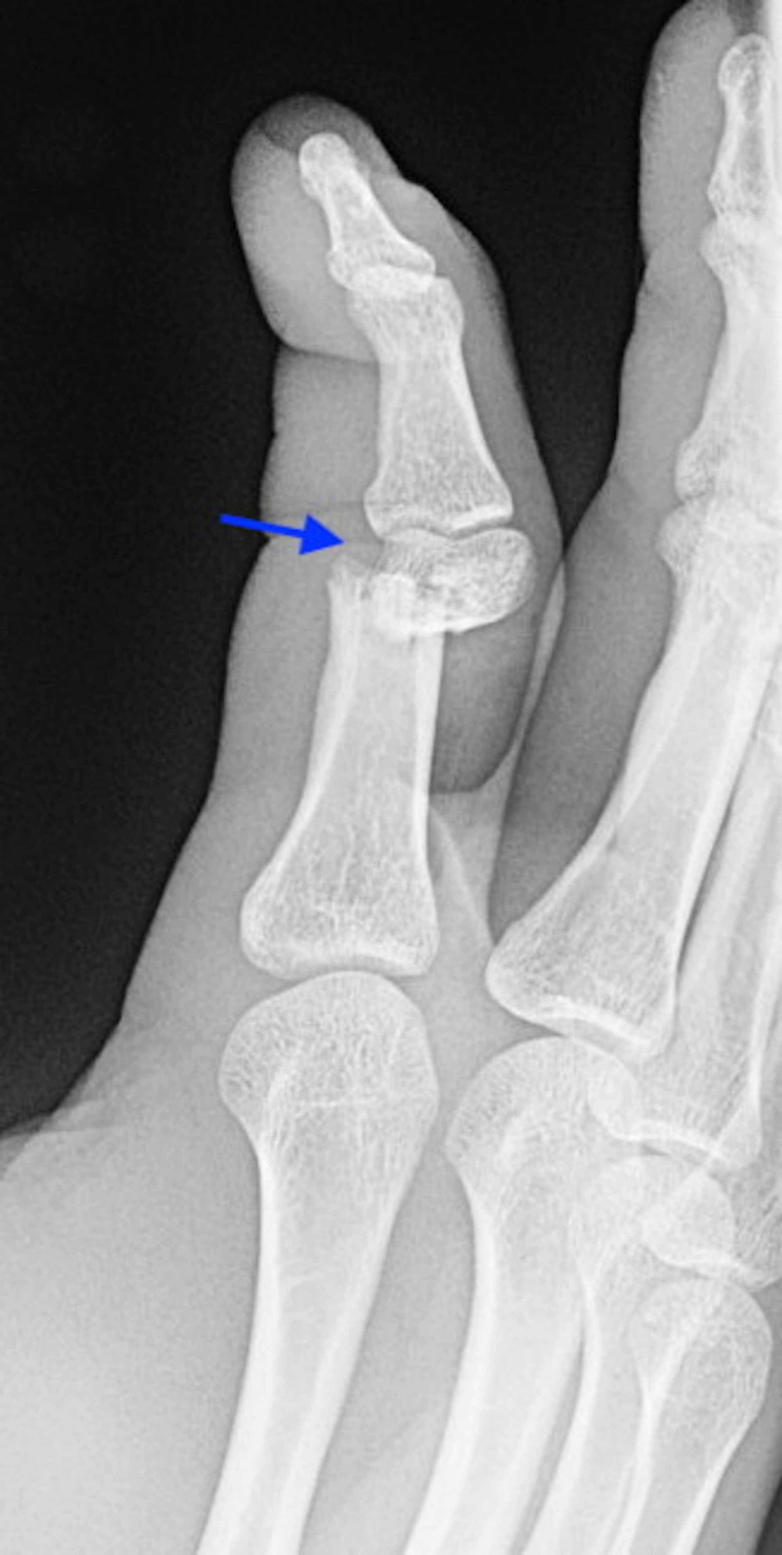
Oblique plain radiograph of the right index finger showing ventral dislocation (arrow) of the proximal metacarpal relative to the distal portion
